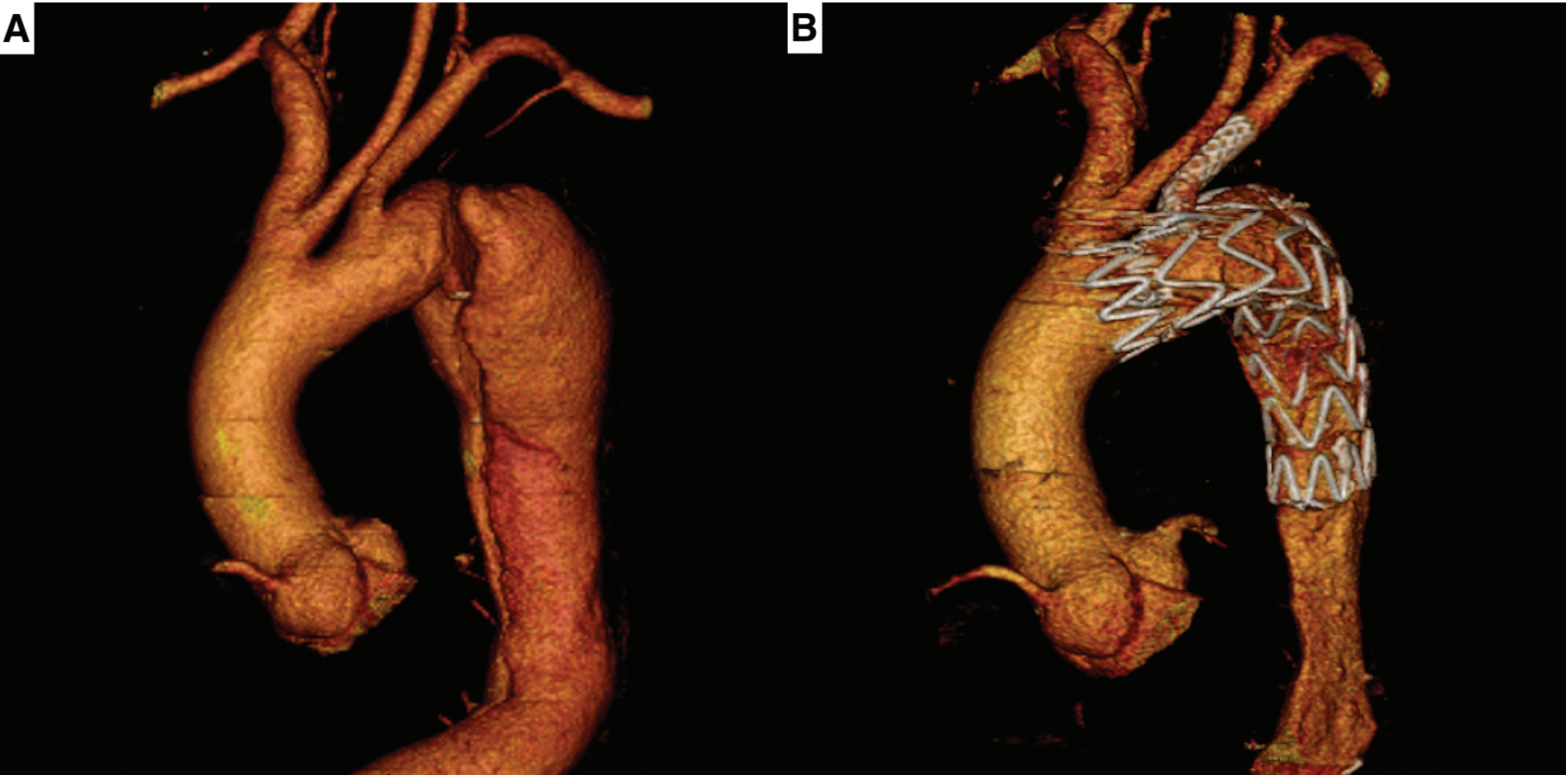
Three-dimensional computed tomography reconstructions show the preoperative image of a Type B aortic dissection beginning close to the left subclavian artery (
